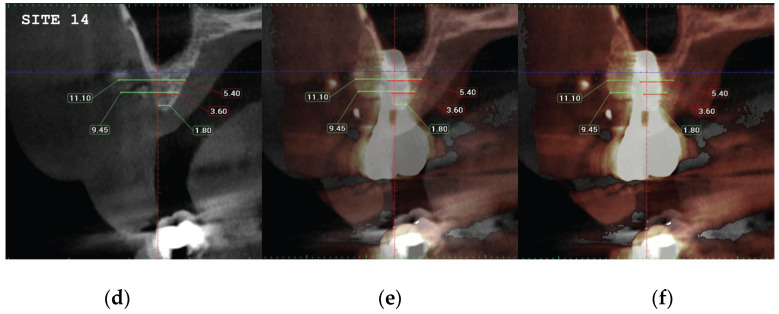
(a) The T1 CBCT image of site 13, (b) the superposed images T1–T2, (c) and the post-image T2, with measurements overlaid. (d–f) Similar setup for the site of premolar 14.
